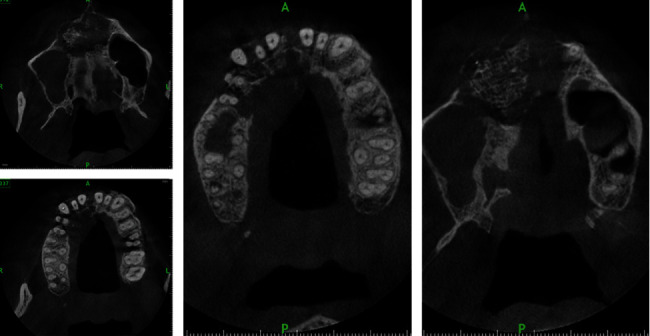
CBCT axial views of case 2 showing gross osteolytic changes with bony sequestrum with haziness on the right maxillary sinus.
